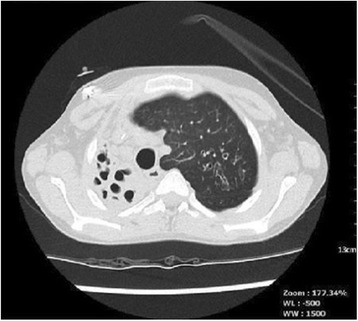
Pre-operative chest high-resolution computed tomography scan showing severe bronchiectasis of the right lung and hyper-expansion of the left lung with mediastinal shift to the right, but relatively minor bronchectasis
